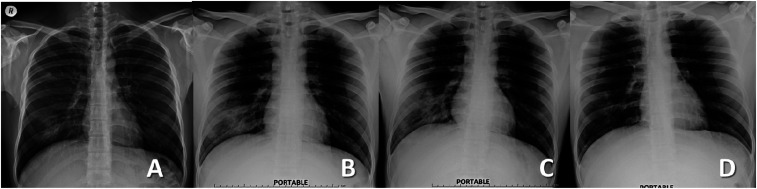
Chest radiography on days 3 (A), 5 (B), 11 (C), and 19 (D) of illness.
